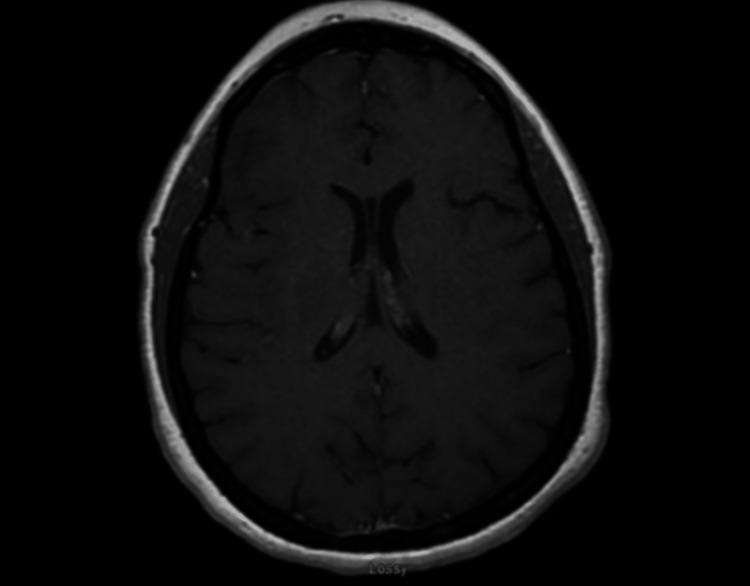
Preoperative T1 axial magnetic resonance imaging of the brain. Normal brain parenchyma and ventricles without hydrocephalus or mass lesions.
